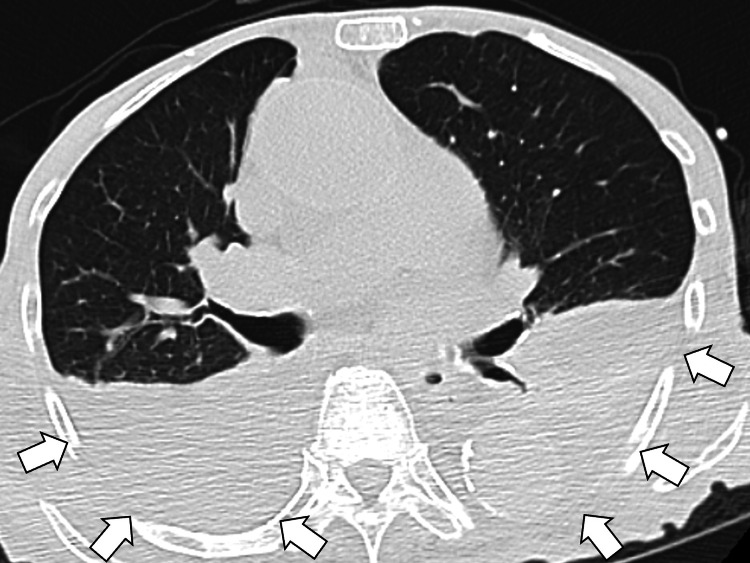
Computed tomography showing no gastrointestinal malignancy nor lymphadenopathy with bilateral pleural effusion (white arrows)
